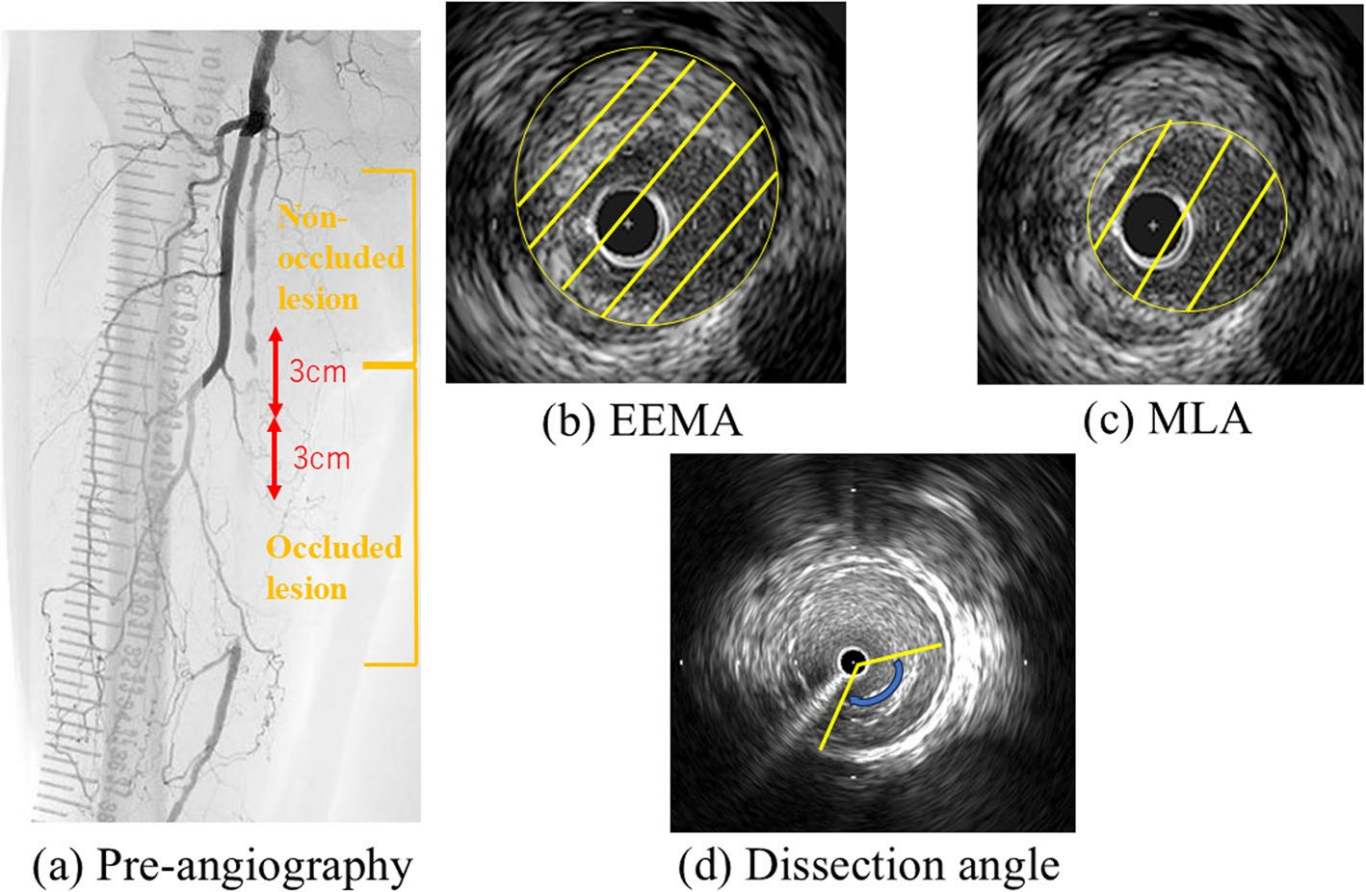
a Pre-angiography. IVUS images were analyzed at 3-cm intervals. b IVUS image and measurement of EEMA. c IVUS image and measurement of MLA. d IVUS image and measurement of dissection angle. IVUS, intravascular ultrasound; EEMA, external elastic membrane area; MLA, minimum lumen area
